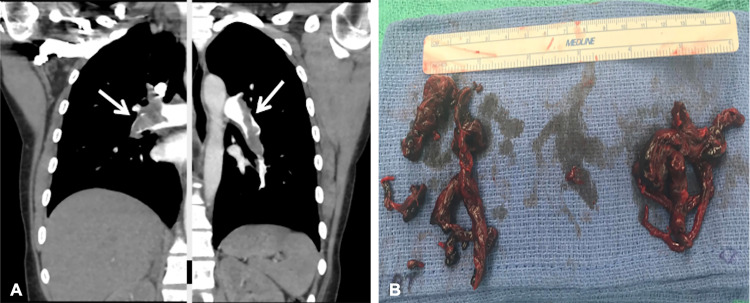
(A) CT pulmonary angiography demonstrating bilateral pulmonary emboli in the main left and right PA (arrows). (B) Thrombi removed during surgical embolectomy. CT computed tomography; PA pulmonary artery
